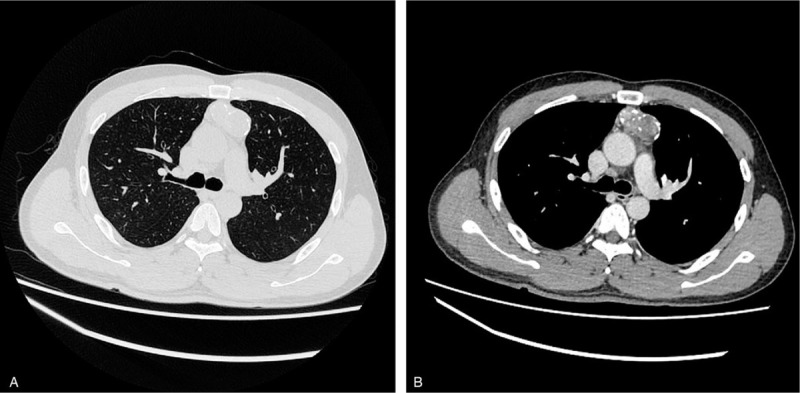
Computed tomography scan of the chest. Computed tomography scan revealed a single, lobulated, well circumscribed, round mass, with an uneven density in front of the pulmonary conus in the anterior mediastinum. The mass was approximately 4.0 cm × 2.2 cm in size, with a smooth outline and multiple calcifications at the edge.
